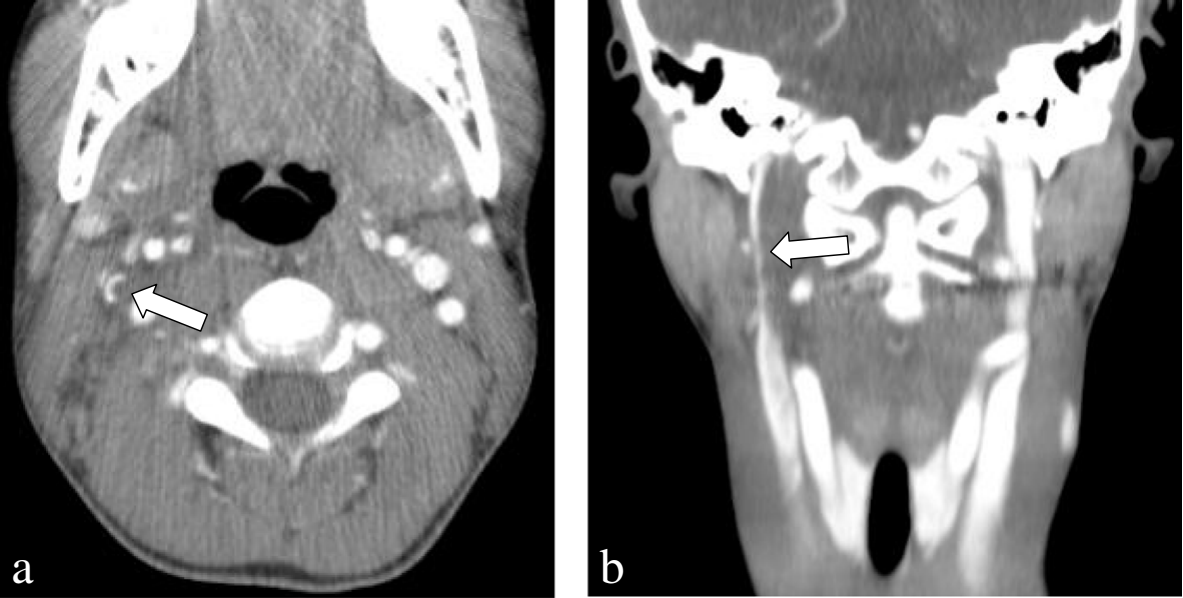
Computed tomography of the neck with intravenous contrast demonstrating partial occlusion of the right internal jugular vein (
